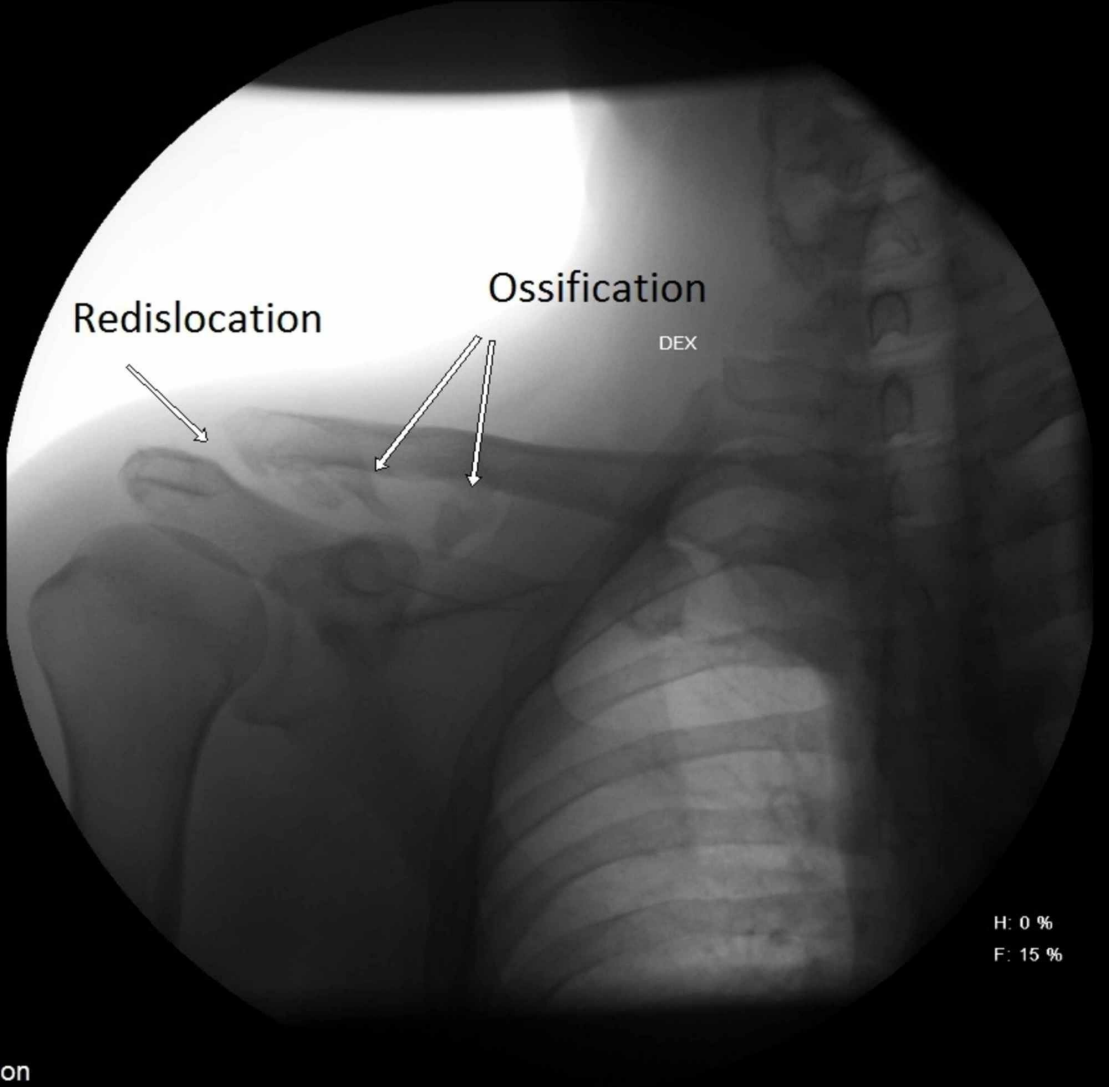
A radiograph of a 40-year-old male patient 20 months after ACJ reconstruction with suture loop. Recurrent dislocation of the clavicle and CC space ossification are present. ACJ, acromioclavicular joint; CC, coracoclavicular
